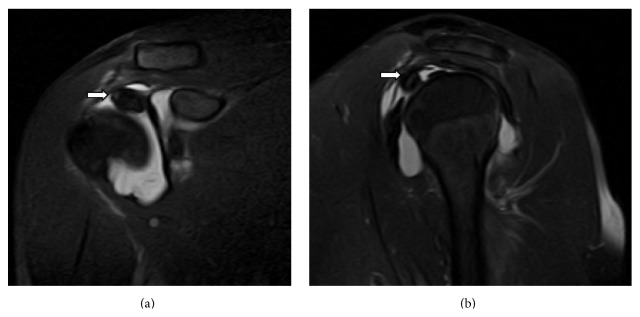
MR imaging of osteochondroma: coronal (a) and sagittal (b) T2 TSE SPIR images.
